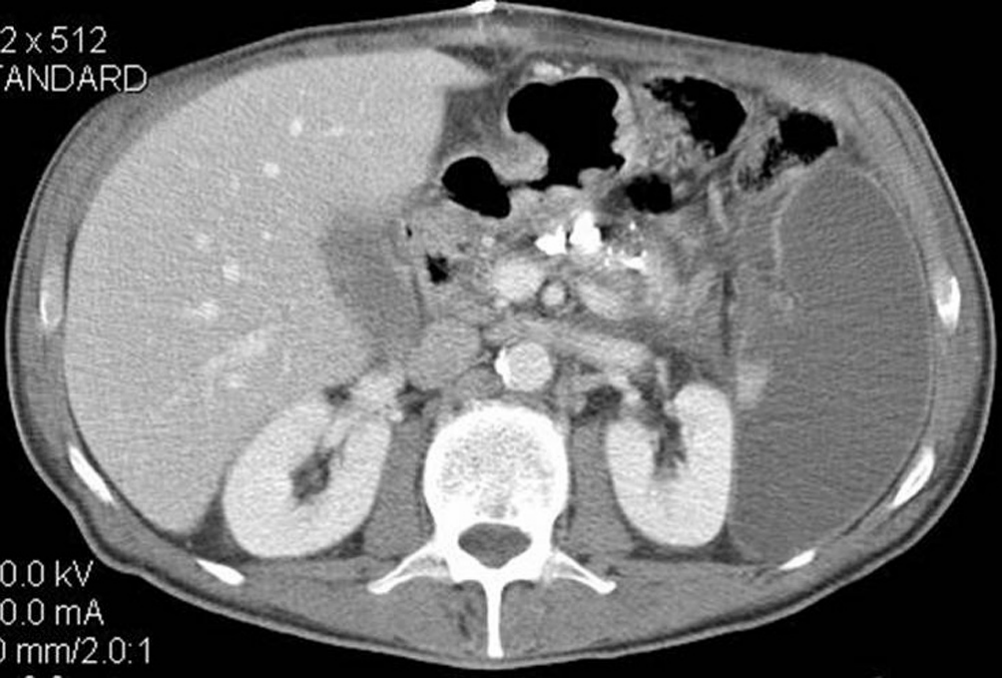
Two weeks post-operative CT scan: in the venous phase the splenic artery is no longer visible; there is hypodensity of the spleen due to reduced vascular supply. Follow-up examinations demonstrated healing of the spleen.
